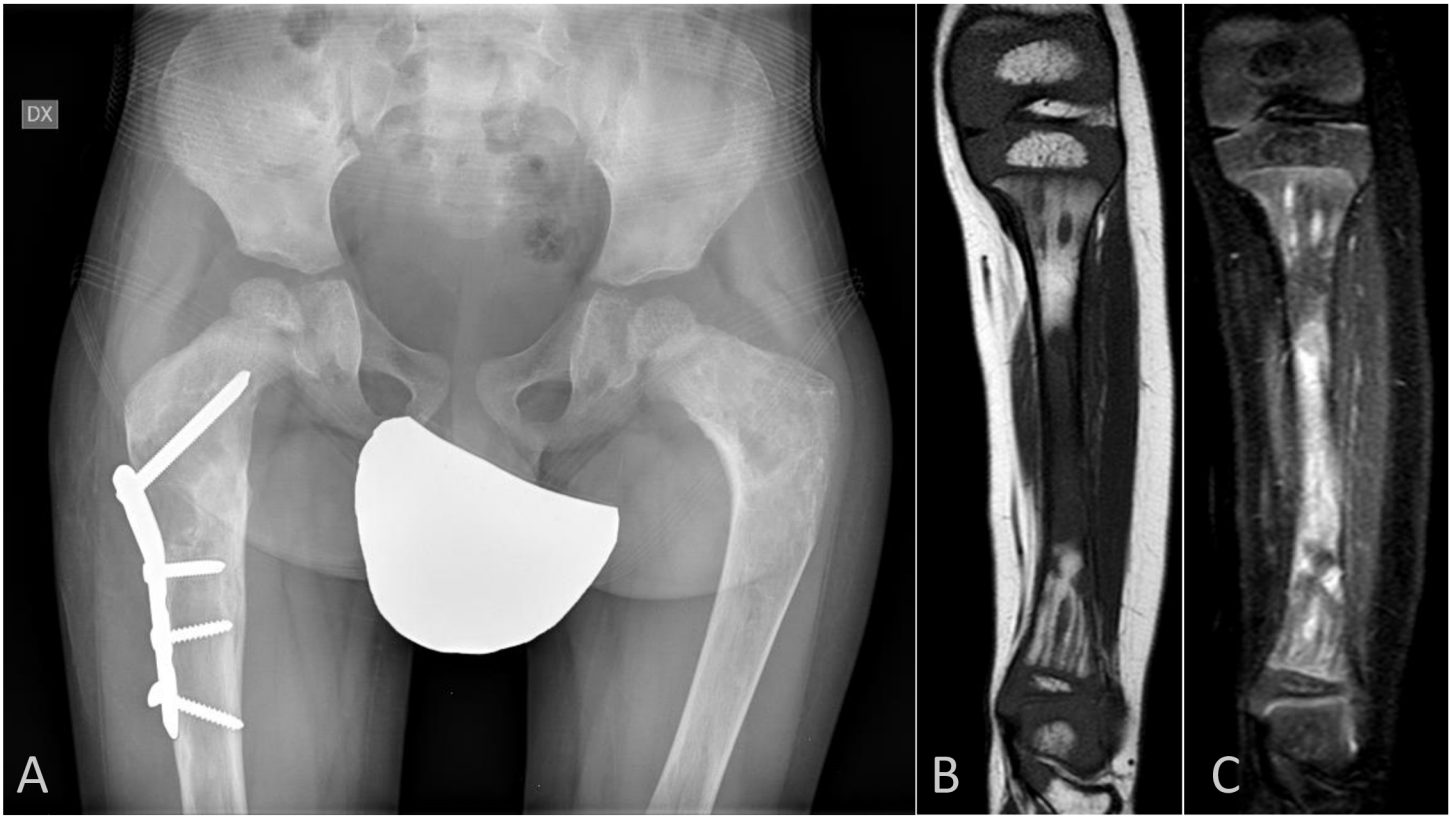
(A) sacrum X-ray performed at the age of 40 months; typical femoral neck enlargement (“sheperd’s crook”) associated with FD can be observed on both sides. (B, C) tibial MRI performed at the age of 33 months; in the image (B) signal hyperintensity associated with FD can be observed in the T1 sequence, especially in tibial diaphysis; in the image (C) the same section shows discontinuous signal hyperintensity in the short tau inversion recovery (STIR) sequence.
